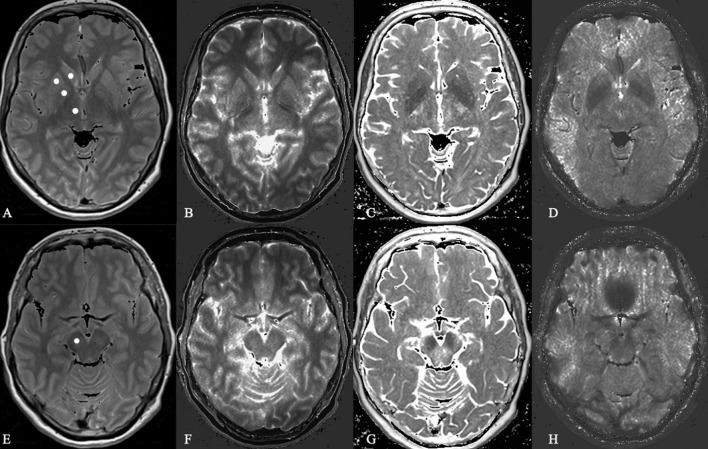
Microstructural analysis of proton density, T1 relaxation time, T2 and T2 prime in a Parkinson’s disease patient. In this Figure shows the basal ganglia (A–D) and mesencephalon (E–H) in proton density-weighted (A,E), T1-weighted (B,F), T2-weighted (C,G), and T2 prime-weighted (D,H) MR sequences obtained from a 62-year-old female PD patient.
